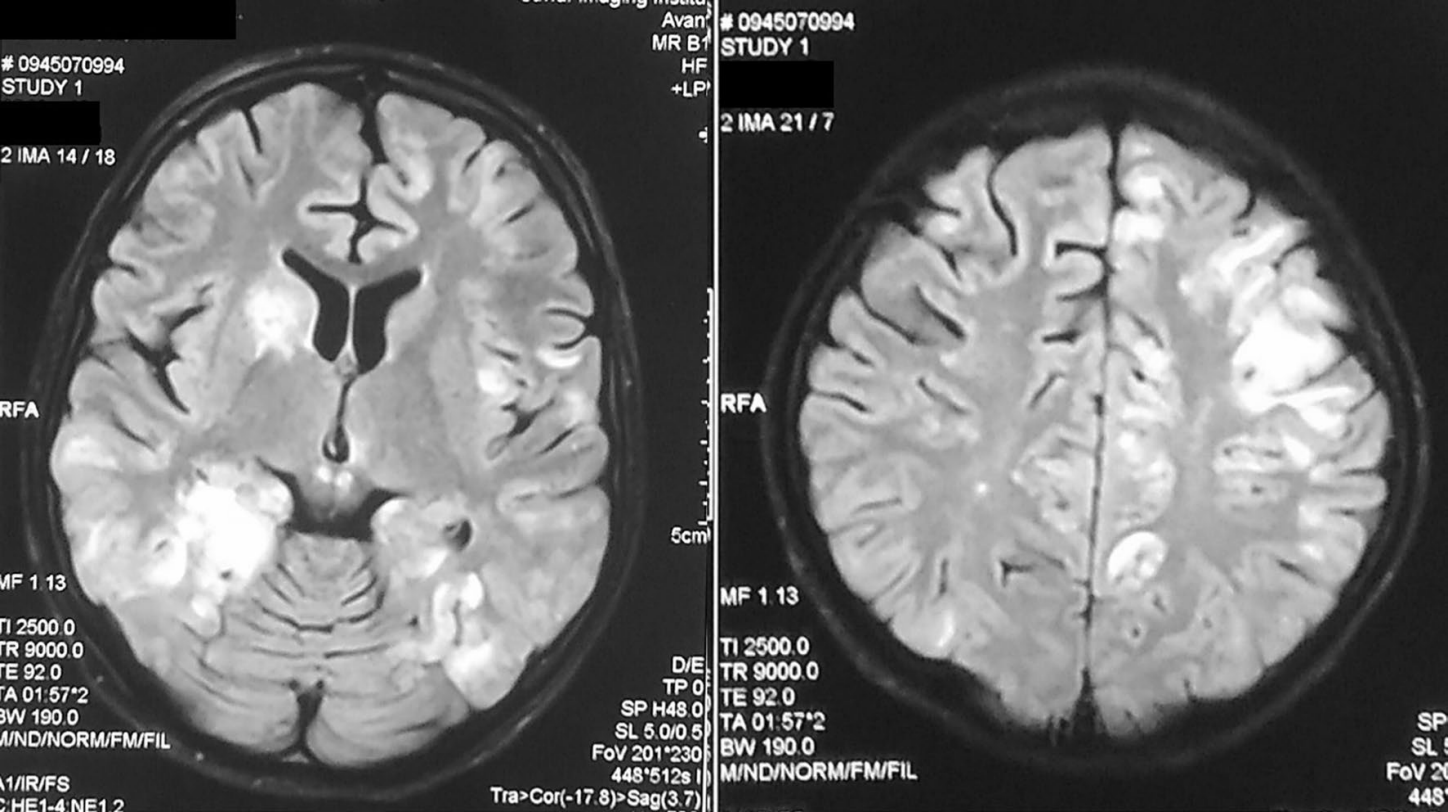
T2 MRI imaging presenting high signals in the cortical and subcortical area (right) and in the caudate nucleus and midbrain (left)
